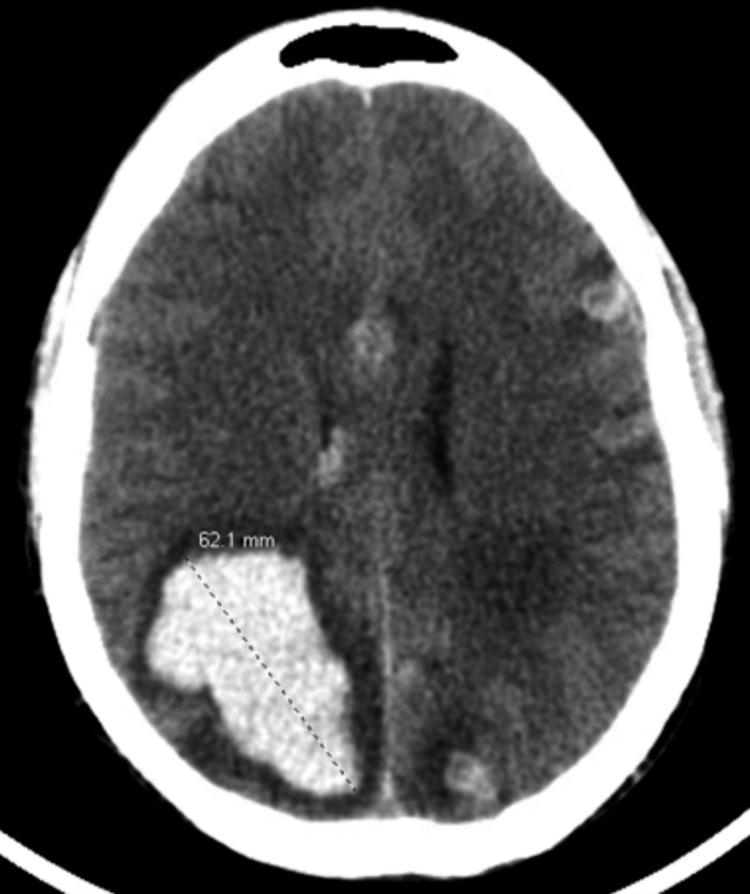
CT brain without contrast with new right intraventricular hemorrhage and multiple hemorrhagic metastases in bilateral cerebral hemispheres. Largest hemorrhagic lesion measuring about 6.2 cm in the right parietal lobe, increased size from prior imaging. Lesions demonstrating perifocal/vasogenic edema. CT, computerized tomography
